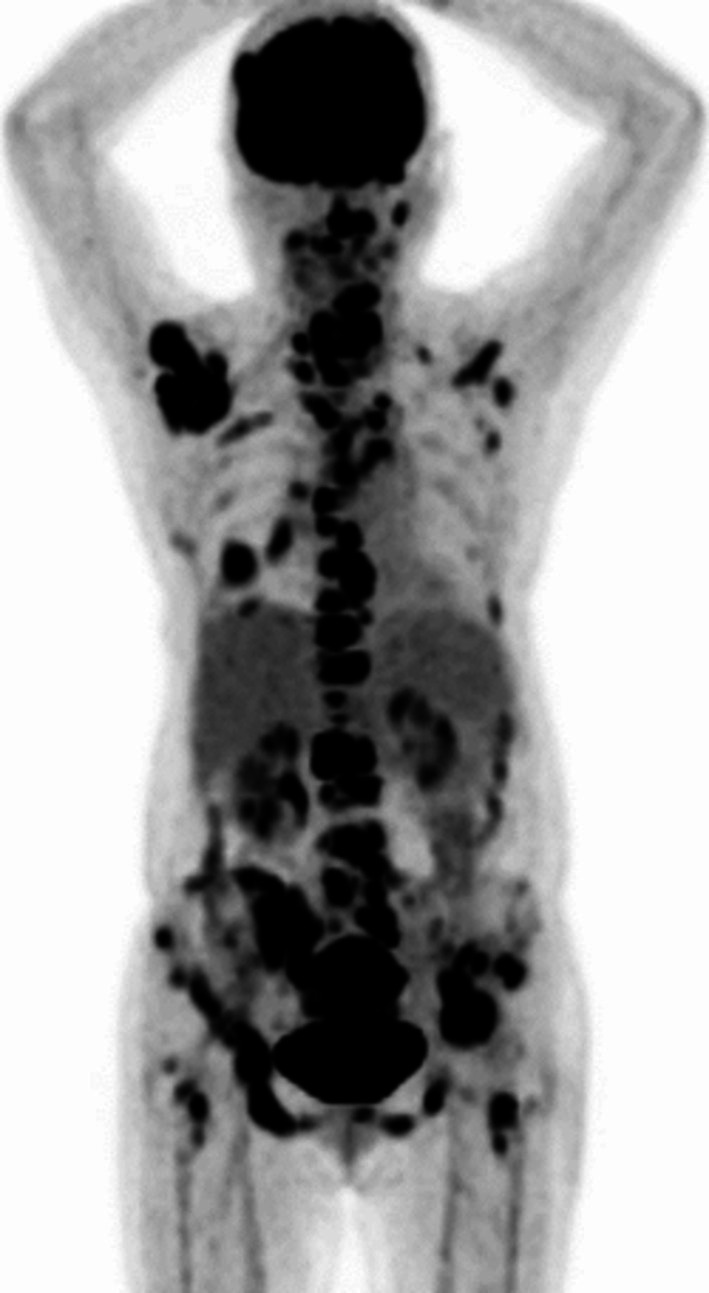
Positron emission tomography‐computed tomography examination showing fluorodeoxyglucose accumulation in the right lung mass, lymph nodes, bone and thyroid gland.
